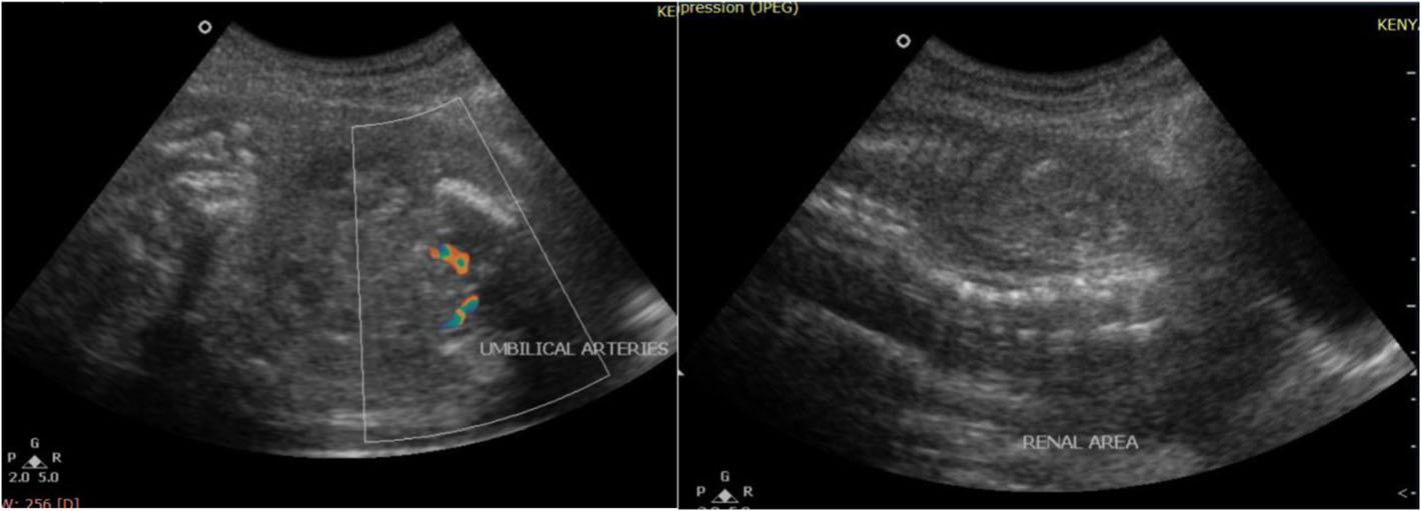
These are images from a mother in the age range 25–29 years Gravida 3 at 31 weeks who presented with a history of fundal height not corresponding to dates. On imaging, both kidneys and the urinary bladder were absent. The thoracic diameter was relatively small likely due to pulmonary hypoplasia and associated oligohydramnios. A diagnosis of bilateral renal agenesis was made. Ultrasonic age corresponded to 25 weeks
